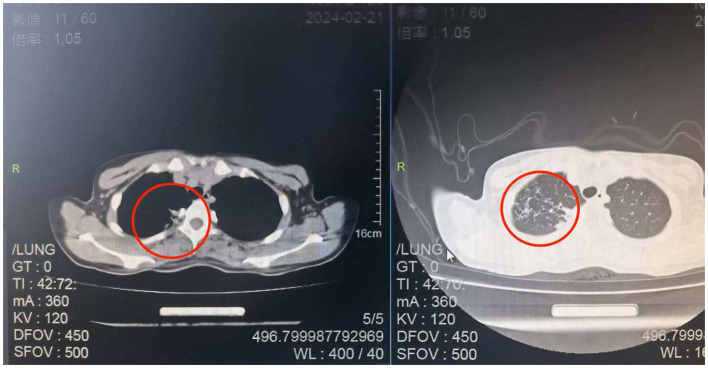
Chest CT before treatment showing patchy and nodular opacities in the right upper lobe.
